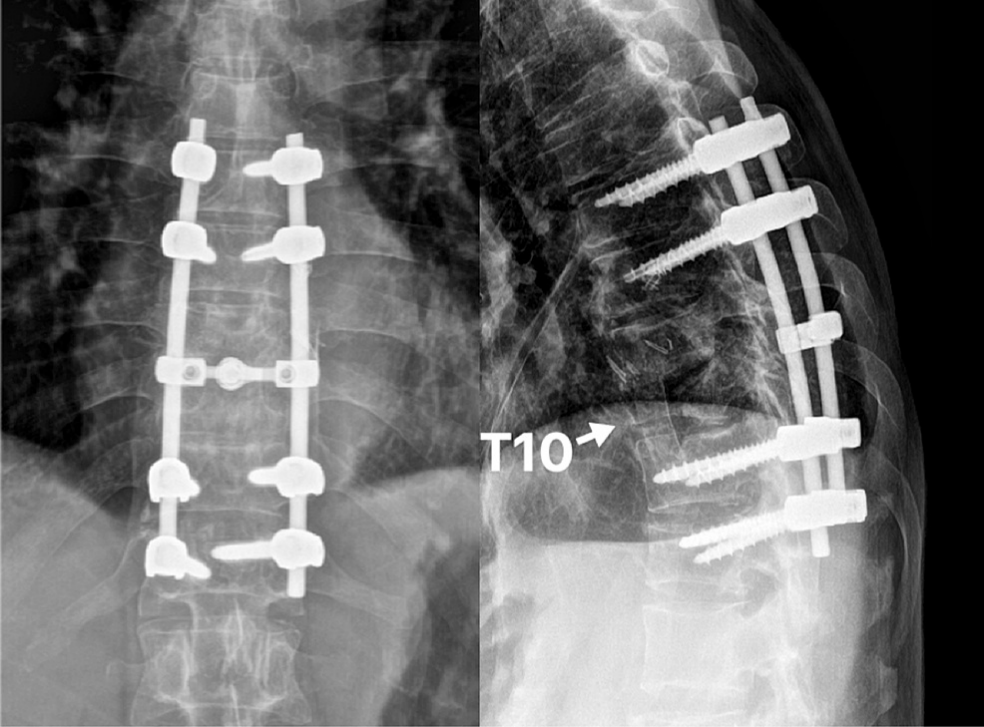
Postoperative spine radiograph.
